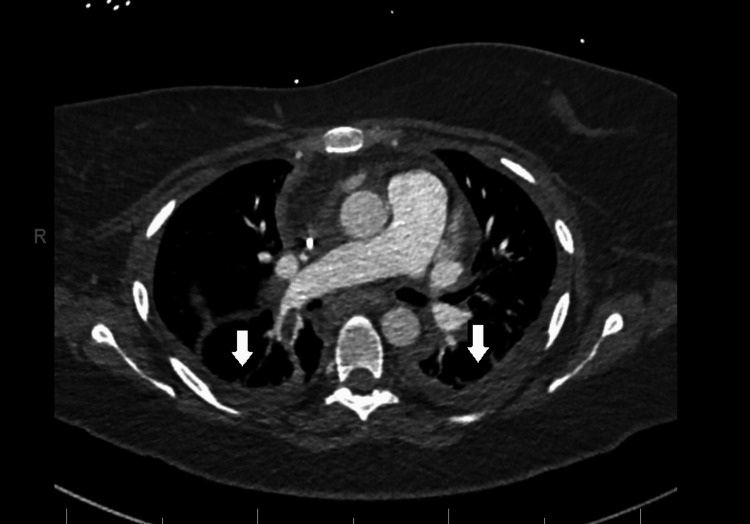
Computed tomography imaging of the chest with arrows depicting reduced bilateral effusions.
